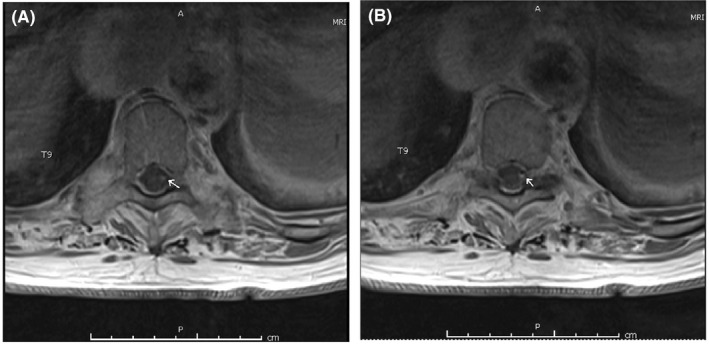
(A–B). MRI with contrast revealing question of focal contrast enhancement at the area in contact with the intrathecal pain pump catheter tip
